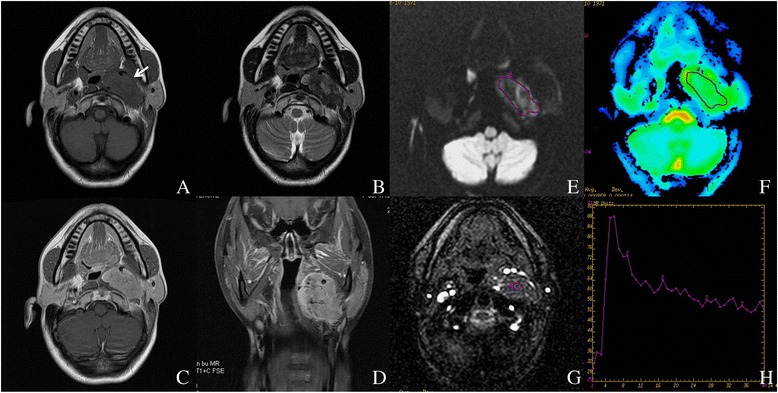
Left carotid body tumor in a 38-year-old female. a, b Non-enhanced axial T1W and T2W images. c Contrast enhanced axial T1W image. d Contrast enhanced coronal T1W image with fat suppression. e, f ROI on DWI (*b* value = 1000 s/mm^2^) and corresponding ADC map. The average ADC value of this lesion was 0.928 × 10^−3^ mm^2^/s. g, h DCE-MRI and corresponding TIC, which showed a washout pattern
